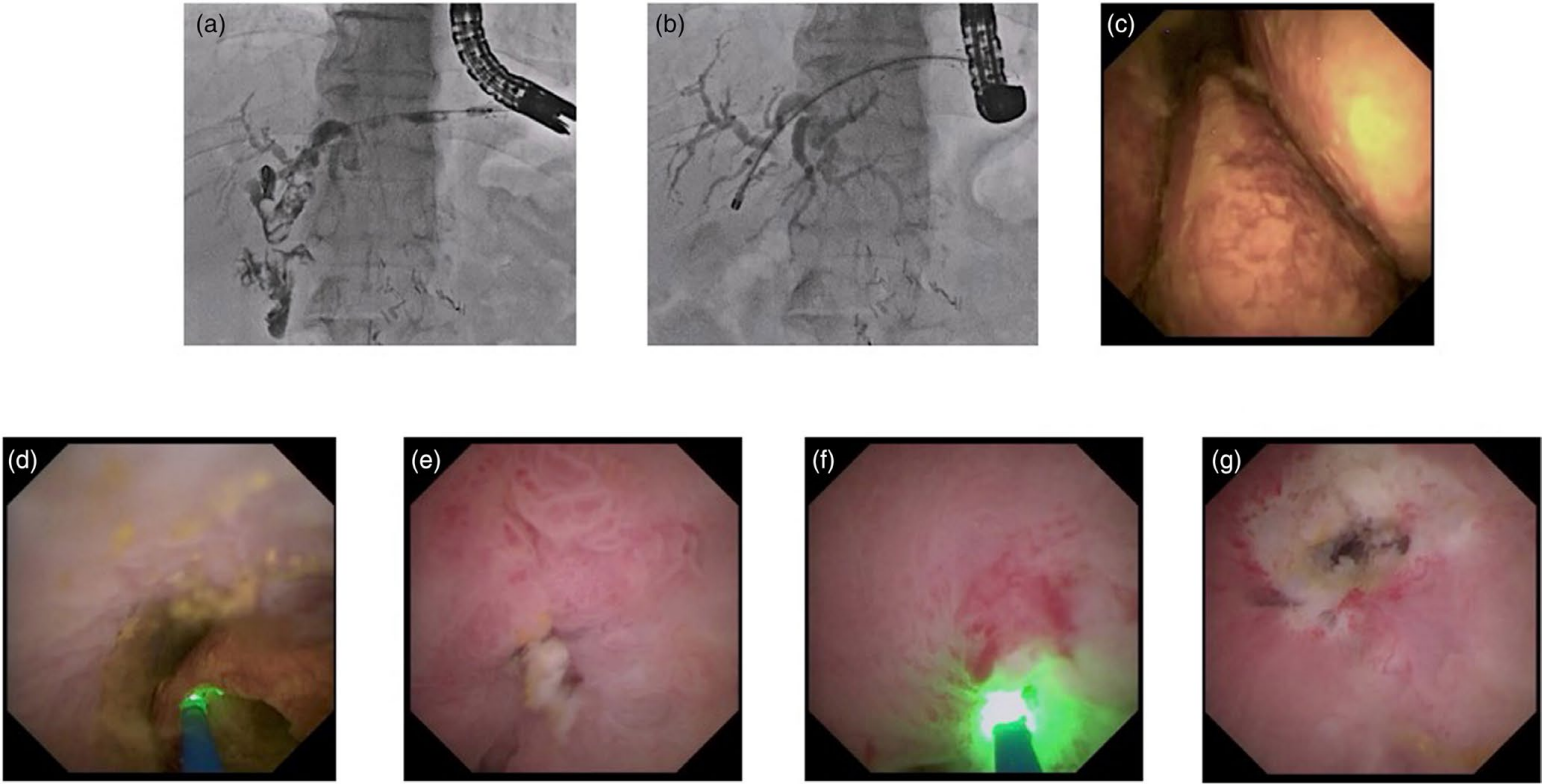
Transluminal cholangioscopy. (a) Cholangiography via the endoscopic ultrasound‐guided hepaticogastrostomy (EUS‐HGS) route shows bile duct stones and a hepaticojejunostomy stricture (HJS). (b) Cholangioscope insertion via the EUS‐HGS route is attempted. (c) Bile duct stones are identified. (d) Antegrade laser lithotripsy is performed. (e) HJS is also identified. (f) Antegrade laser ablation for HJS is attempted. (g) Stricture resolution is obtained.
